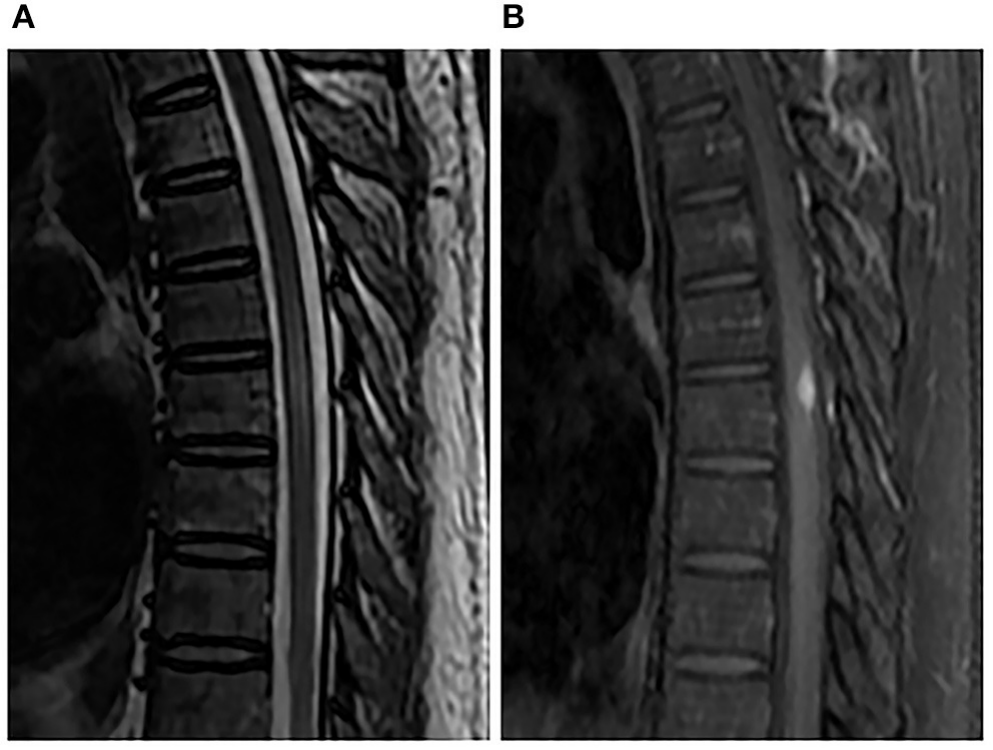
Spinal MRI performed 1 month after penicillin therapy. Sagittal T2-weighted image (A) and enhanced T1-weighted image (B) showed that the nodule and perilesional edema were reduced.
